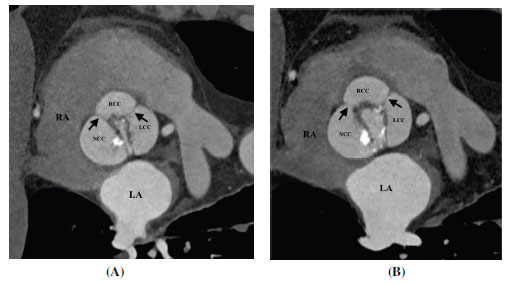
Type 2 bicuspid aortic valve. (A) Axial contrast-enhanced computed tomographic angiography image diastolic frame and (B) Axial contrast-enhanced computed tomographic angiography image systolic frame image showing bicuspid aortic valve with a two thin non-calcified raphe between right-left and right- non-coronary cusps. Abbreviations: RA; right atrium, RVOT; right ventricular outflow tract, LA; left atrium, LV; left ventricle, Ao; ascending aorta, RCC; right coronary cusp, LCC; left coronary cusp, NCC; non-coronary cusp.
